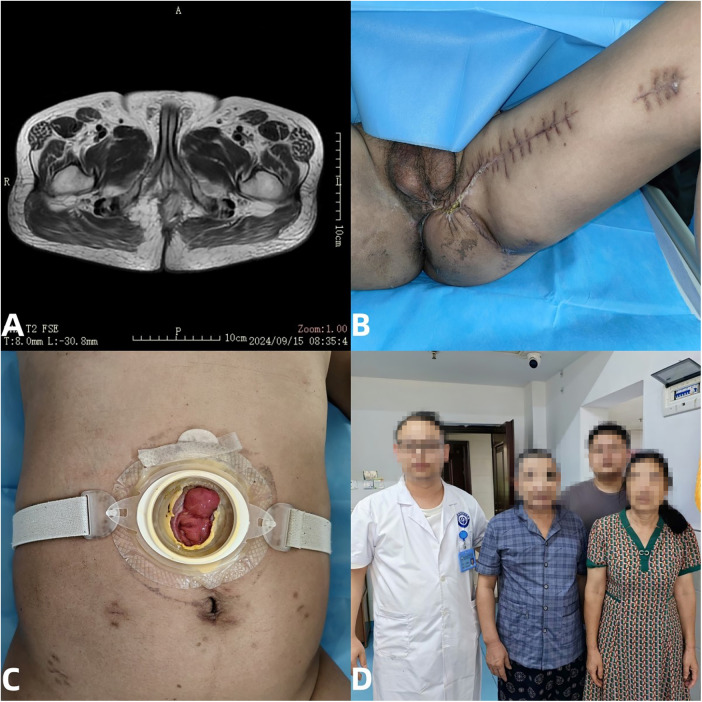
(A) 2-month follow-up after surgery, the pelvic MRI of the patient shows that the perineal rectal resection cavity is completely filled with the gracilis muscle, and there is no residual or recurrent rectal cancer. (B) The patient's perineal area wound completely healed. (C) The abdominal transverse colostomy defecation was good. (D) His daily life was completely self-sufficient, and their weight increased by 5KG compared to the emergency department in May 2024.
